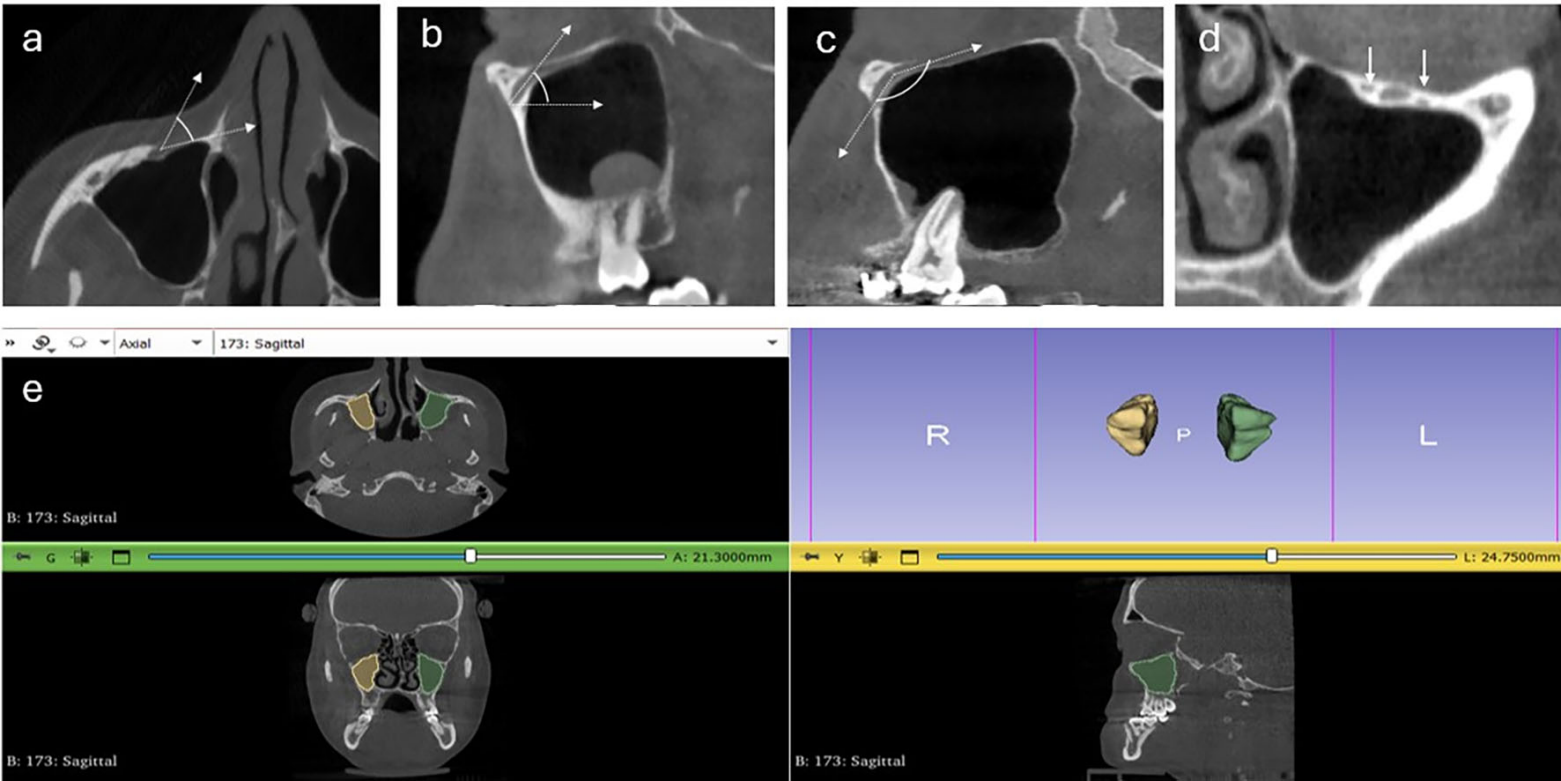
a Axial CBCT image showing infraorbital canal angle (IOCA)1 constructed between the IOC and the horizontal axis, b sagittal CBCT image showing IOCA2 constructed between the IOC and the sagittal axis, c sagittal CBCT image showing IOCA3 constructed between the IOC and infraorbital groove, d coronal CBCT image showing two accessory IOCs, and e three-dimensional reconstruction of maxillary sinus (MS) and volume measurement
